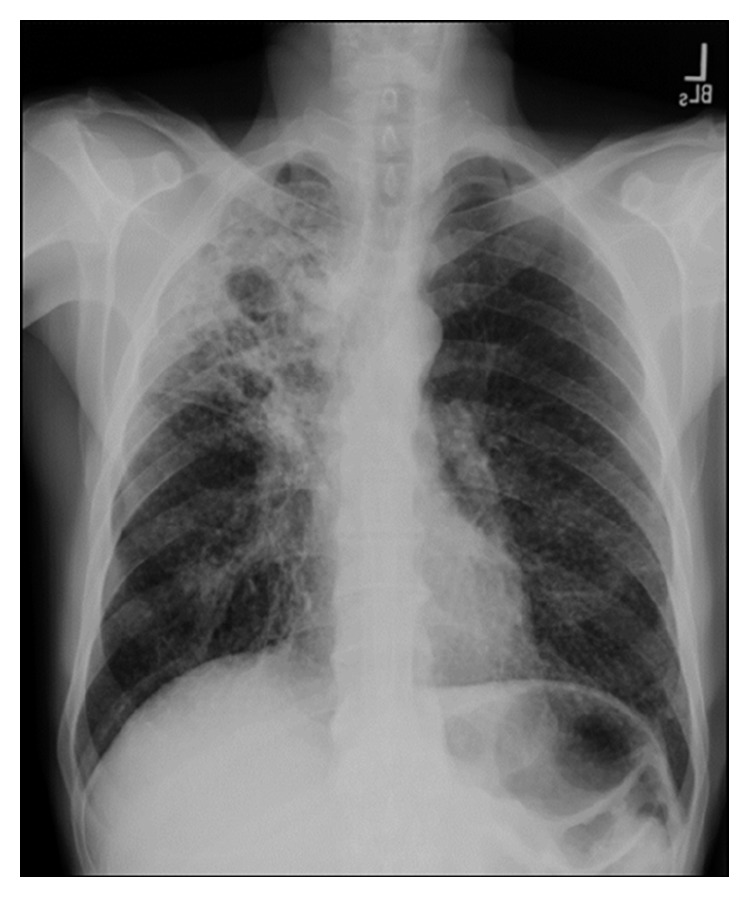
Chest X-ray showing cavitary opacity in the right upper lung field.
